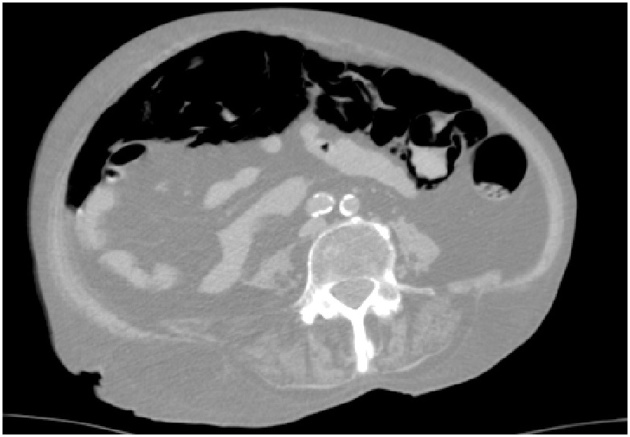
CT scan shows an extensive pneumoperitoneum.
